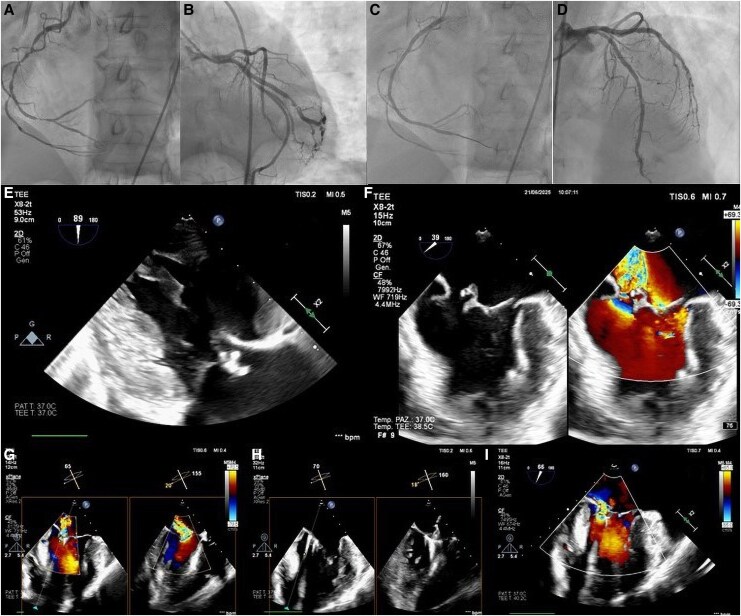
(A) Right coronary angiography with diffuse disease and subocclusion in distal tract. (B) Left coronary angiography with subocclusion of obtuse marginal branch and severe stenosis of proximal left anterior descending artery. (C) Right coronary post-angioplasty results and (D) left coronary post-angioplasty result. (E) TOE in *trans*-gastric view showing a mass attached to the prolapsing leaflet corresponding to postero-medial PM head detachment. (F) TOE bicommissural view showing flail leaflet, posterior leaflet tethering, and massive mitral regurgitation at colour Doppler. (G) A more than moderate residual mitral regurgitation from the antero-medial portion of the valve after the first mitraclip (XTW) implantation. (H) Second mitraclip (NTW) implanted medially to the first one, at the para-commissural A3-P3 scallop level. (I) Final result showing mild/mild-moderate mitral regurgitation, originating between the two clips. TOE, transoesophageal echo; PM, papillary muscle.
